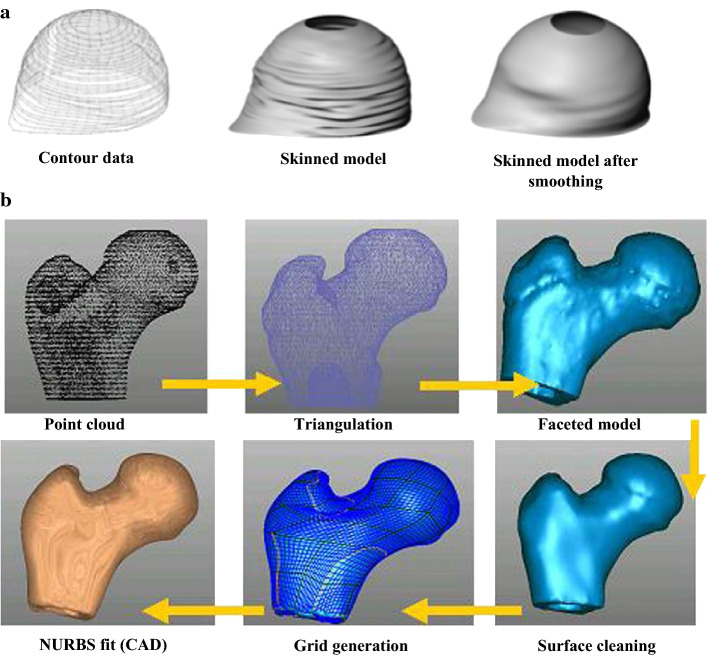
a 3D reconstruction of CT data (reprinted with permission from Springer Nature, J. H. Ryu et al. [23] copyright (2004)), and b reverse engineering approach to provide CAD model (reprinted from Sun et al. [19] Copyright (2005), with permission from Elsevier)
